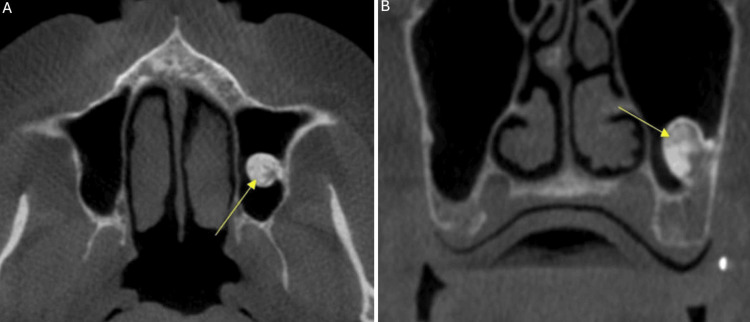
(A) Axial view at the level of the inferior nasal conchae, area of the left maxillary sinus; CBCT acquired in 2023. (B) Coronal view at the level of the missing first and second molars, area of the left maxillary sinus; CBCT acquired in 2023. The yellow arrows show the well-defined non-uniform high-density entity under investigation. CBCT: cone-beam computed tomography
